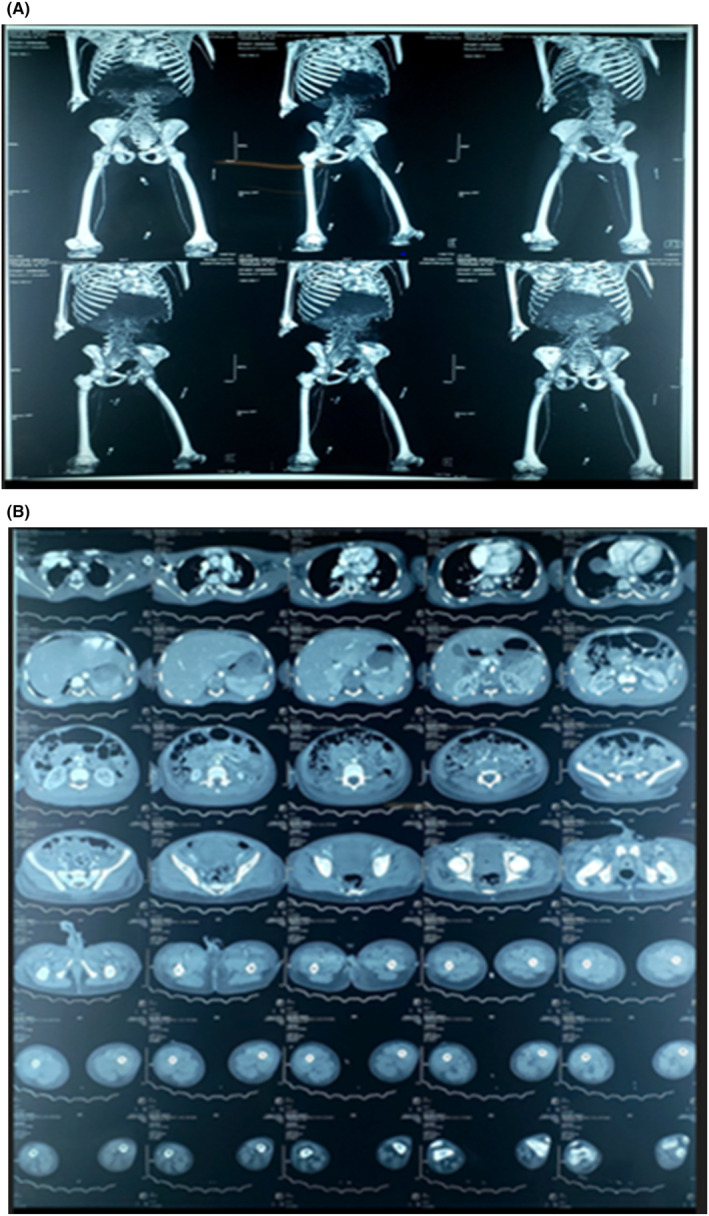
(A) 3D reformats of the aorta and its distributions showing on obstruction. (B) An enhanced helical chest and abdominal CT scan using an angiogram protocol demonstrated well‐sustained contrast enhancement throughout the thoracic and abdominal aorta, with no evidence of linear filling defects, mural thrombi, or atherosclerotic plaques. The aorta exhibited normal diameters at all assessed levels.
